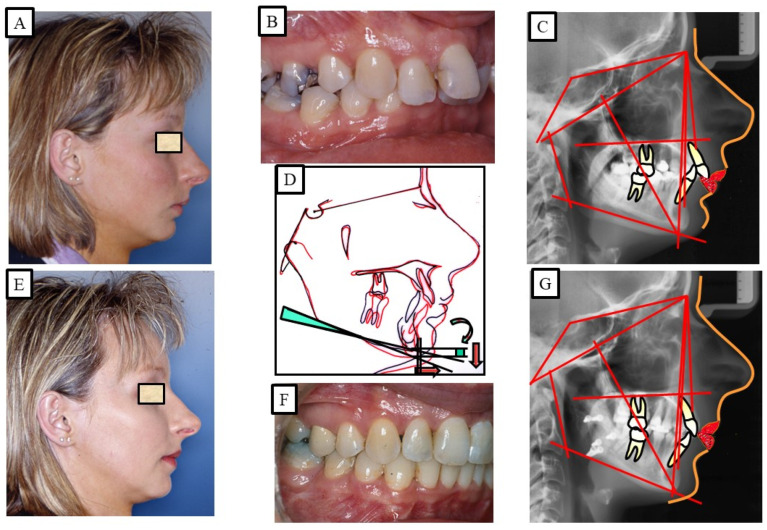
An adult patient with Class II dysgnathy and deep bite (skeletal). The correction of skeletal Class II and the skeletal deep bite is performed via combined orthodontic surgical treatment. Posterior rotation of the mandible during surgical mandibular advancement caused bite elevation and lengthening of the lower facial height. (A–C) show orthodontic surgical treatment. (D) shows the over-positioning of the pre-treatment (black) and post-treatment (red) radiographs due to the changes in sagittal and vertical dimensions. The surgical rotation of the mandible caused the opening of the mandibular angle, which led to the lengthening of the lower facial height. (E–G) show after orthodontic treatment.
